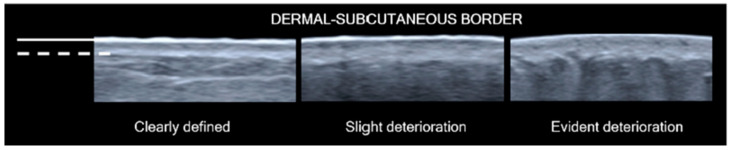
Greyscale ultrasound images of dermal-subcutaneous tissue layer border integrity. Borderlines of the epidermis (solid line) and dermal-subcutaneous border (dotted line) are indicated in white markings. A clearly defined border appears as a bright, uninterrupted hyperechoic line that separates the tissue layers with minor undulation along the border length (left). Slight border clarity deterioration is demarcated by a loss in contrast to echogenicity between tissue layers (middle). Evident deterioration of border integrity with the borderline interrupted by large serrations extending between tissue layers (right).
